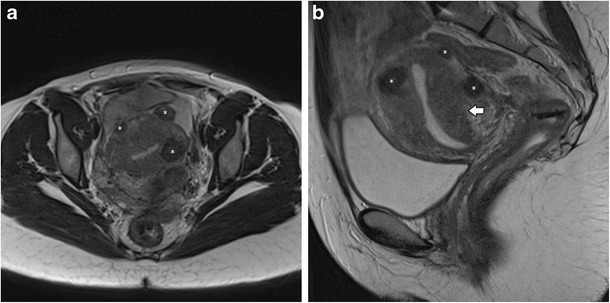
Diffuse adenomyosis and leiomyomas: a Axial T2 and b Sagittal T2-weighted images; diffuse thickening of the junctional zone (white arrow) in relation to diffuse adenomyosis and multiple hypointense masses representing leiomyomas (asterisks)
